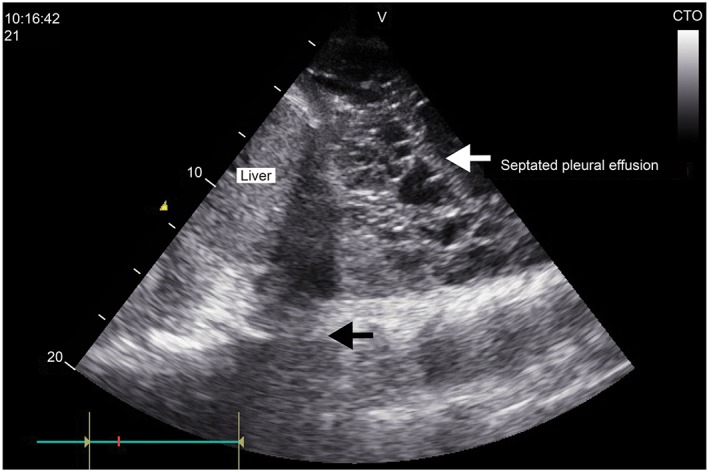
Ultrasound image. Complex pleural effusion with multiple septations. PE pleural effusion
